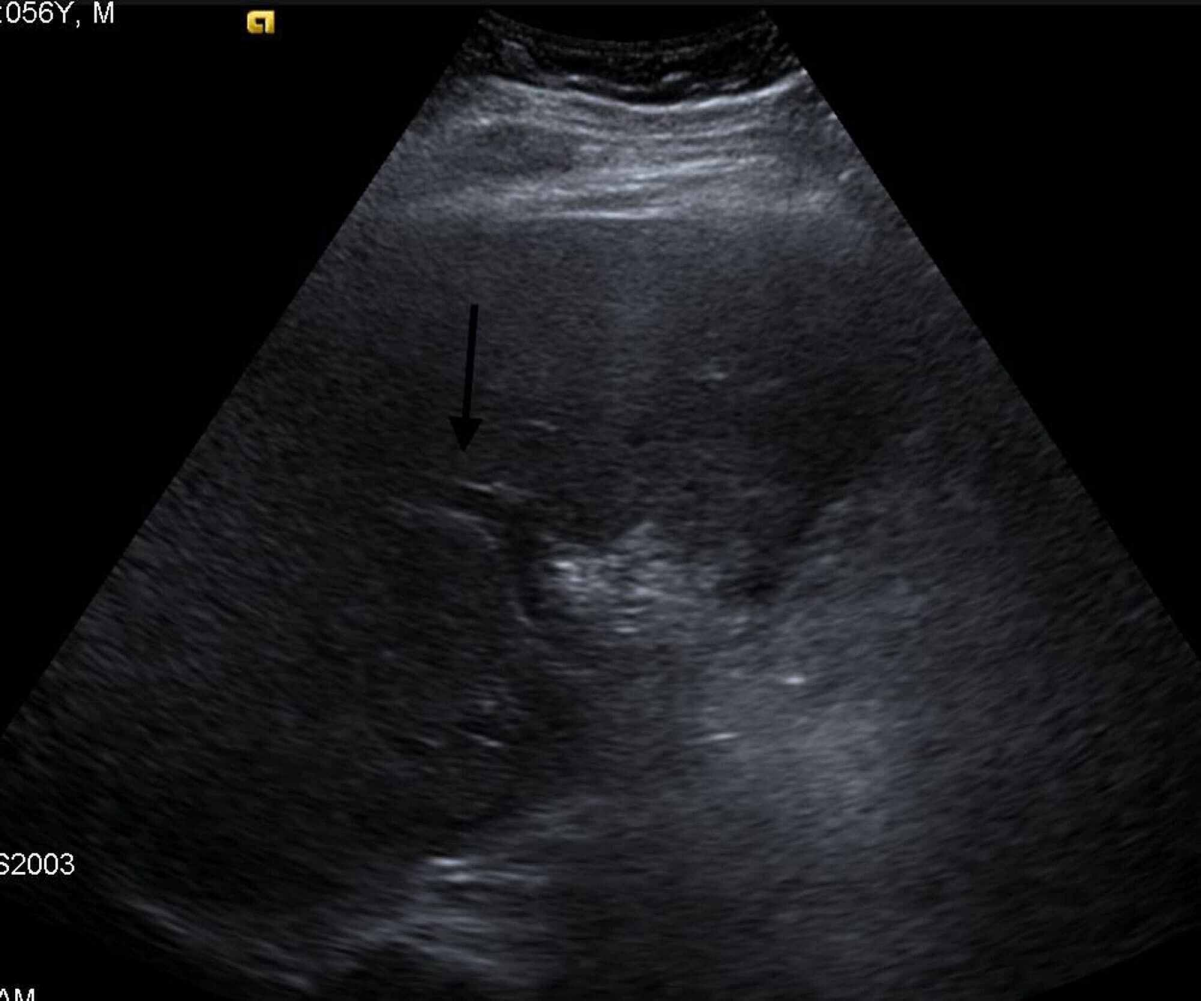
Gallbladder Ultrasound shows no common bile duct dilation
